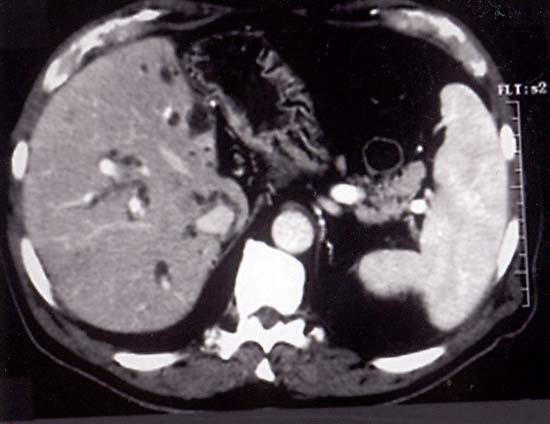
A 58 year old man, who 8 years ago underwent left hepatectomy and cholecistectomy, for complicated intrahepatic biliary stones, presented with jaundice and weight loss. Enhanced CT scan showed marked intrahepatic biliary dilation.
